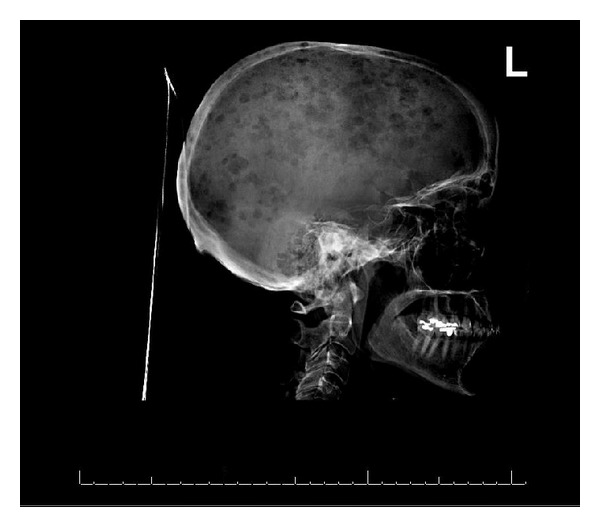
X-ray of the head showing multiple lucent calvarial defects described as lytic lesions.
